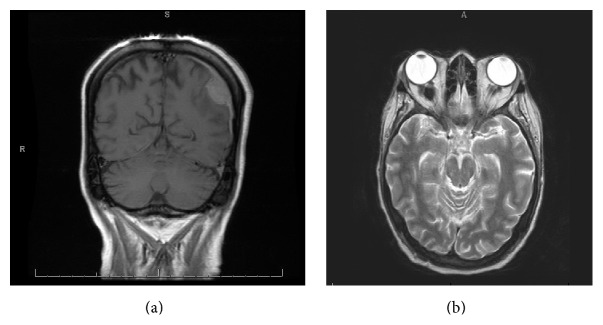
MRI brain coronal section (a) and transverse section (b) showing dural-based mass in the left temporoparietal region as well as mass lesions within the orbits.
